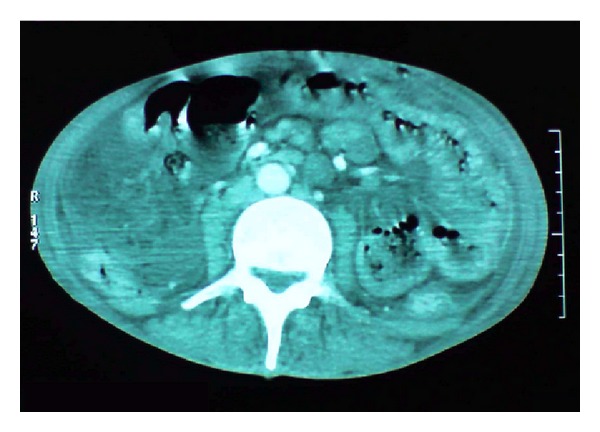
The contrast-enhanced computed tomography scan of the abdominal and pelvic region showed multiple mesenteric and retroperitoneal necrotic adenopathies.
